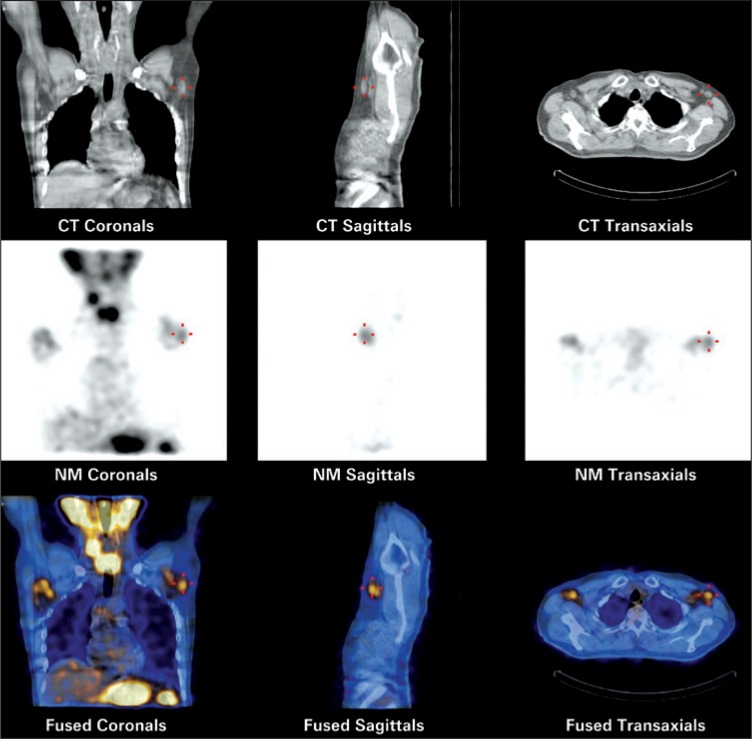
SPECT/CT 4 days after I-131 therapy. Beside radioiodine concentration in the surgical remnants of the thyroidectomy, significant uptake is detected in the axillary lymph nodes, mainly in the left axillat axilla
